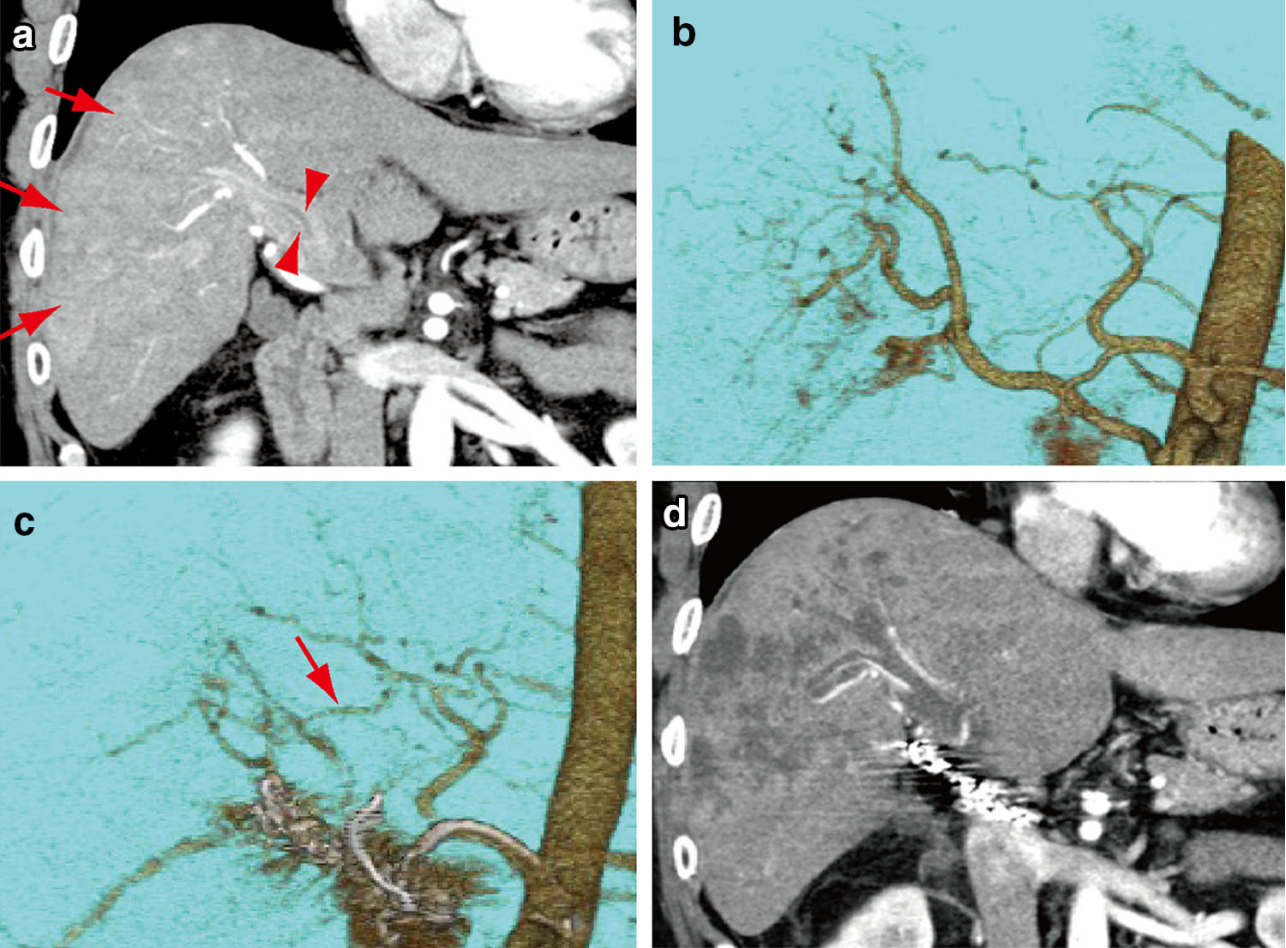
CT findings for case 1. a A coronal image in the arterial-dominant phase demonstrates diffuse HCC (*arrows*) with PVTT (*arrowheads*). b 3D-reconstructed CT angiography shows RHA branches from the SMA. c 3D-reconstructed CT angiography after port-catheter system implantation shows a communicating vessel (*arrow*) between the LHA and the RHA. d A coronal image in arterial-dominant phase demonstrates a marked decrease in vascularity of both parenchymal tumors and the PVTT one day after the procedure
